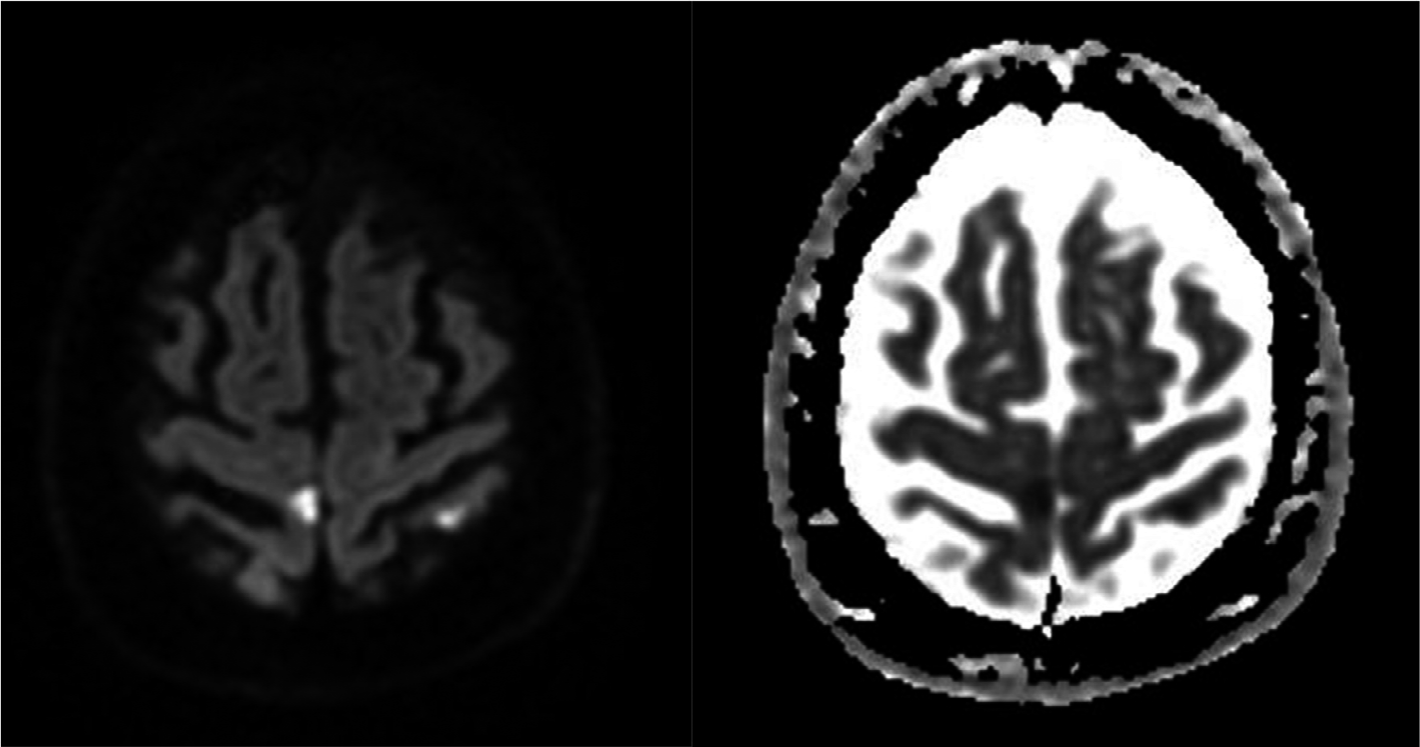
DWI bright/ADC dark signal suggests an acute infarct in the right paracentral lobule, likely embolic, which corresponds to the left toe weakness.
